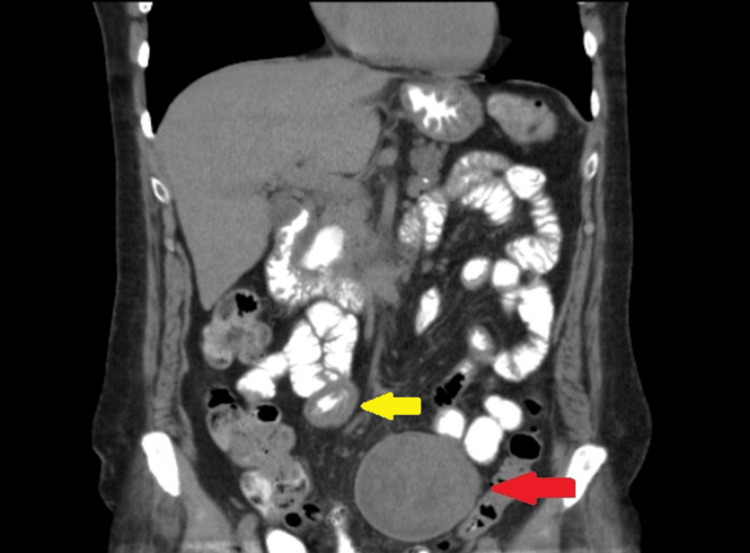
The CT scan (coronal view) showing a lesion (red arrow) and bowel wall thickening (yellow arrow).
